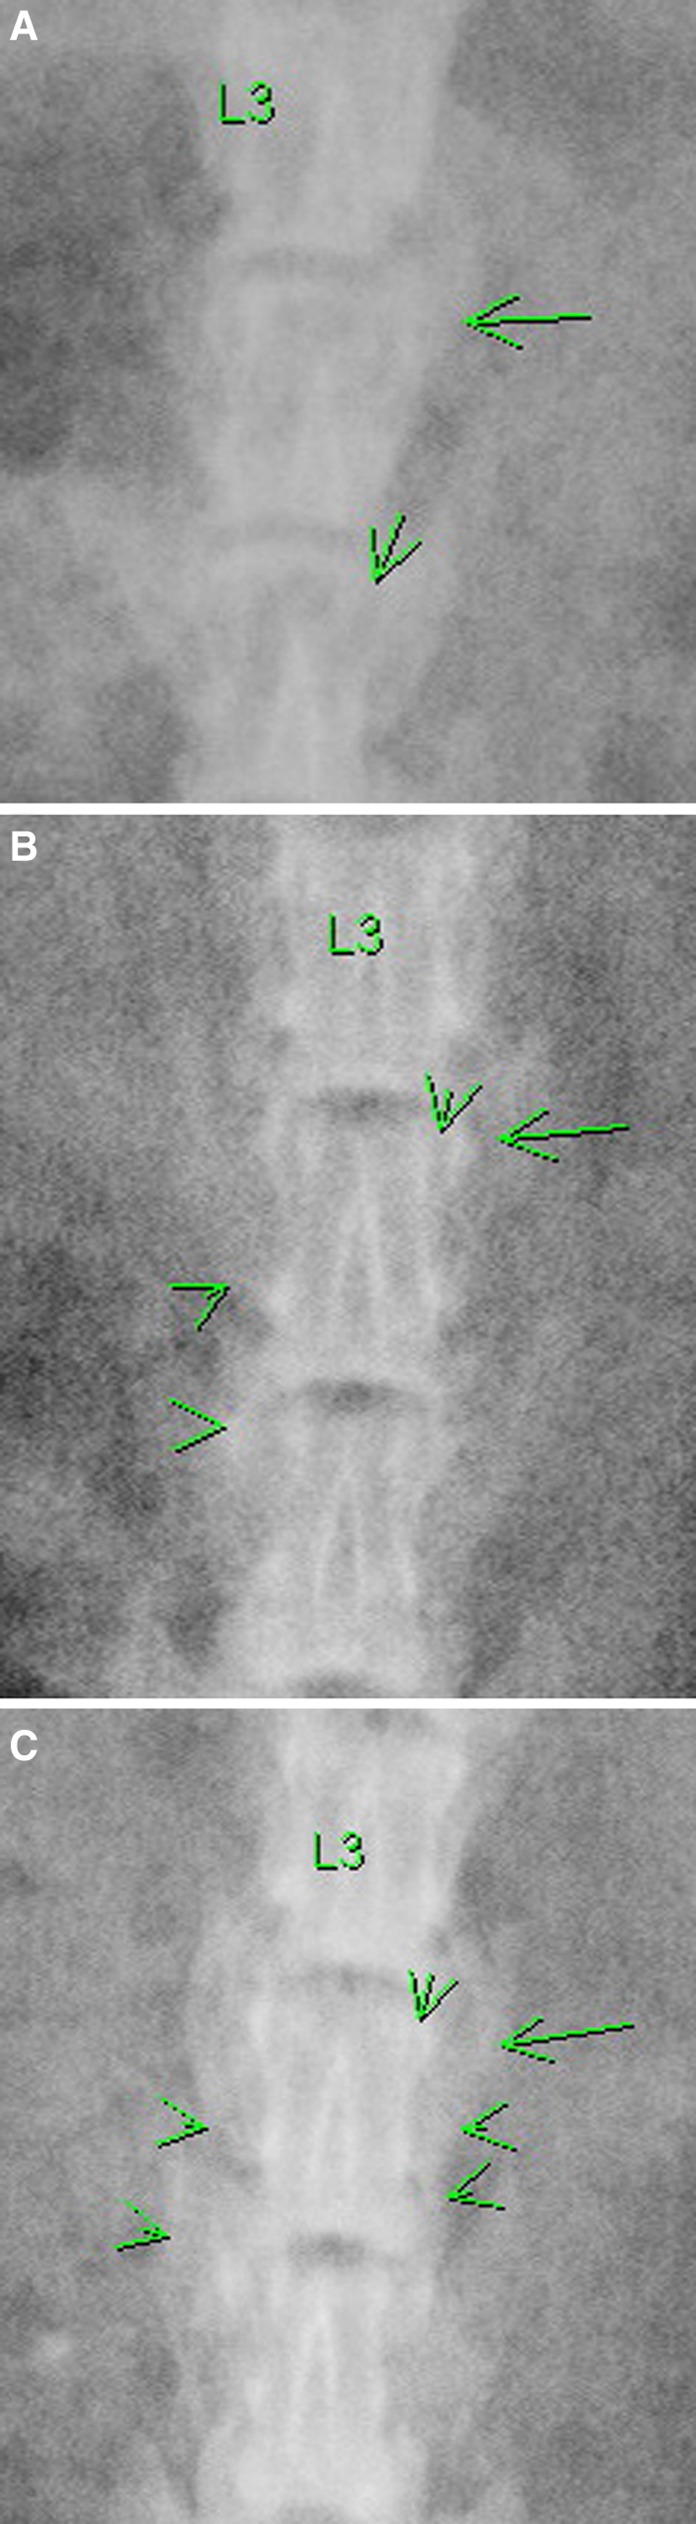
The processes transversus (*long arrow*), facet joint (*short arrow*), fused bony graft (*arrow head*) of lumbar area are *highlighted with arrows*. Typical radiographs of a non-fusion, b partial fusion, and c good fusion are presented
